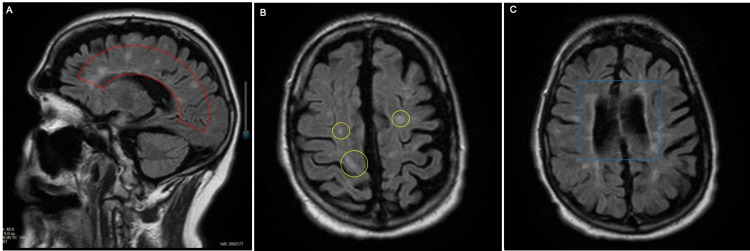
T2-weighted MRI images of the brain. A: Sagittal view showing radiographic findings of Dawson’s fingers in multiple sclerosis (red arc). B: Transverse view with new lesions (yellow circles). C: Transverse view with paraventricular lesions (blue box).
